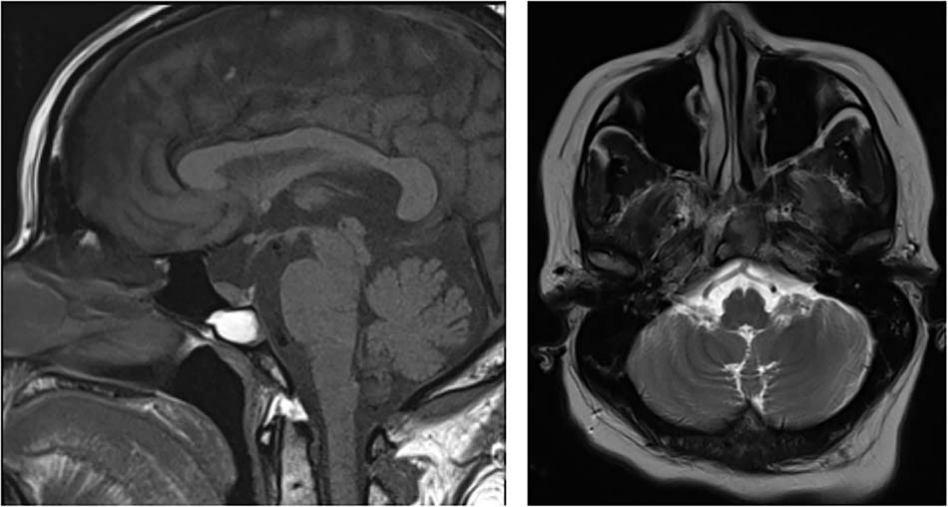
MRI Pituitary (Sagittal T1 weighted image [T1WI] and Axial T2WI) showing the mucocele (high T1WI, low T2WI signal content) and, now, dehiscence at the posterior clival cortex.
